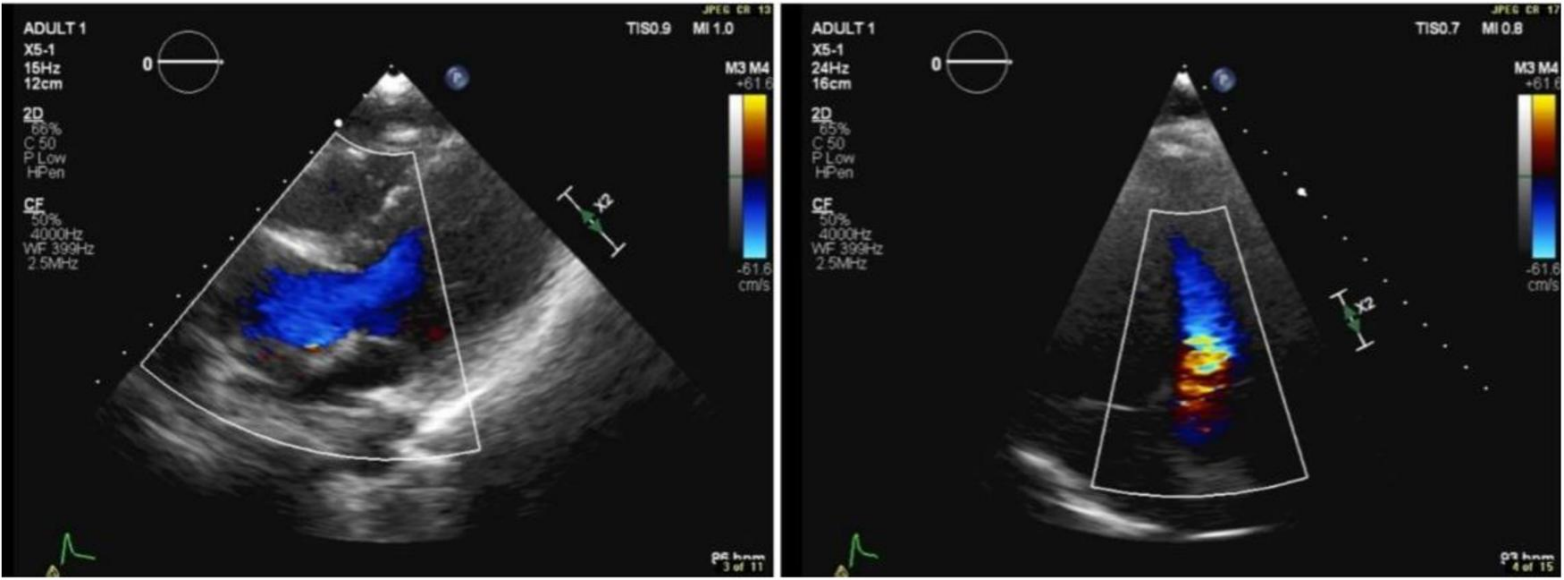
Transthoracic echocardiographic color Doppler showing flow turbulence through the RV-PA conduit. Peak gradient: 25 mmHg; mean gradient: 13 mmHg.
